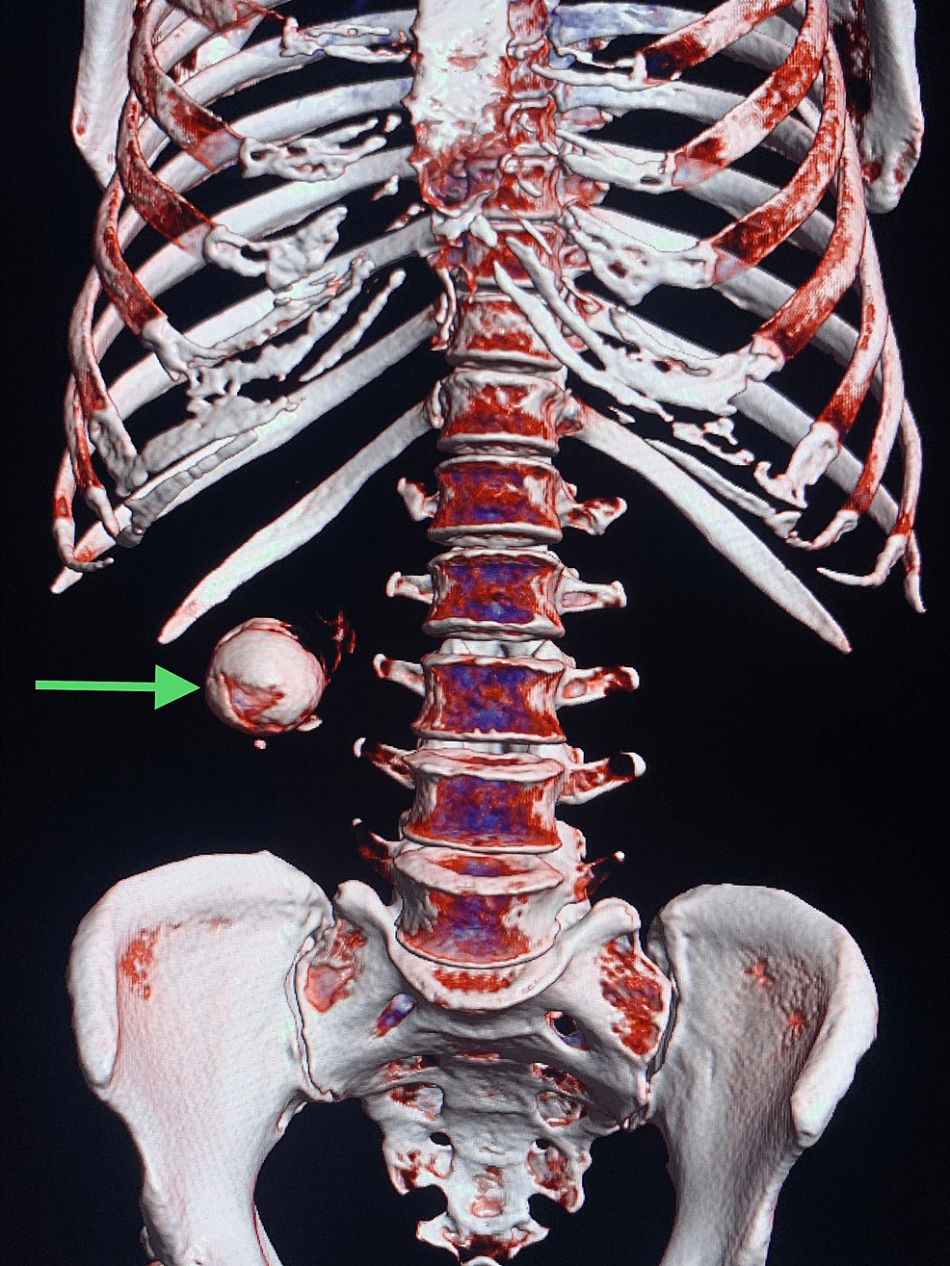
Reconstructed three-dimensional CT image showing a giant gallstone. CT: computerized tomography.
